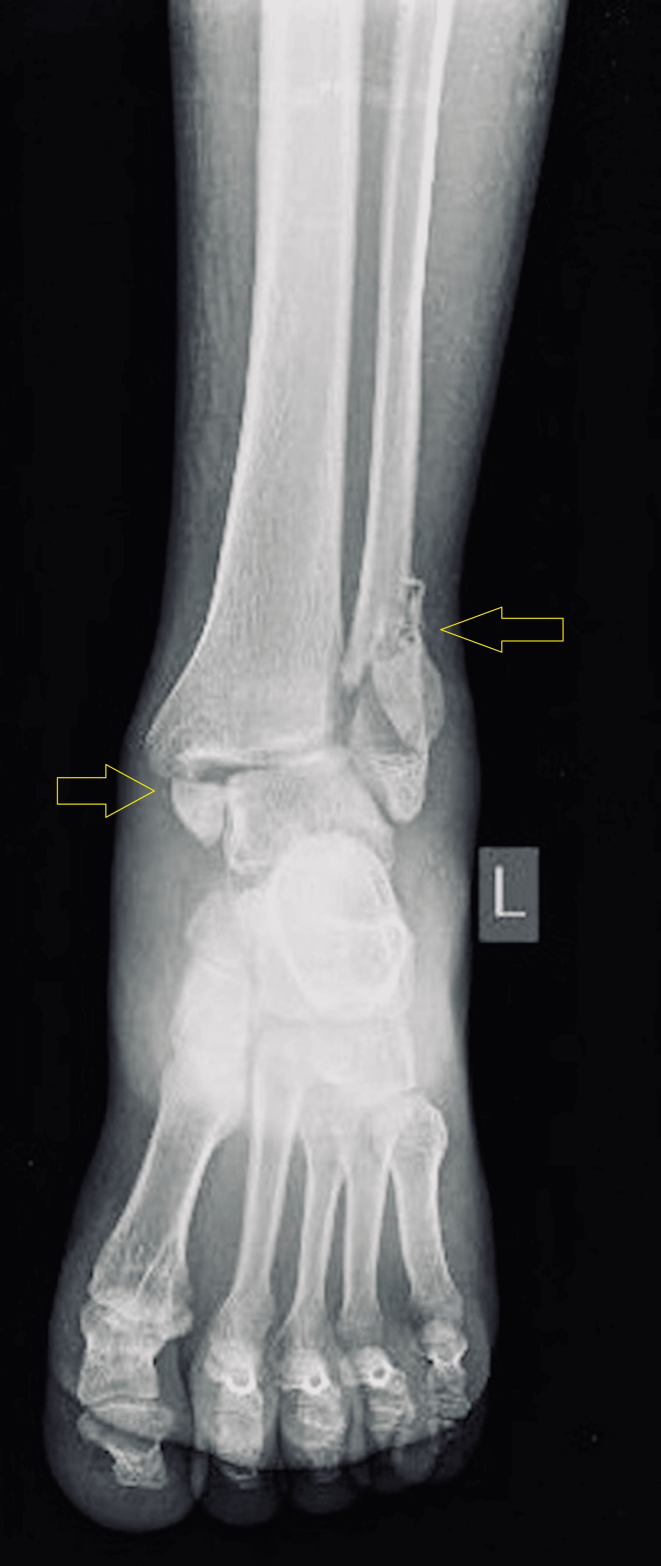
Pre-operative X-ray (anteroposterior (AP) view) of the left ankle.
